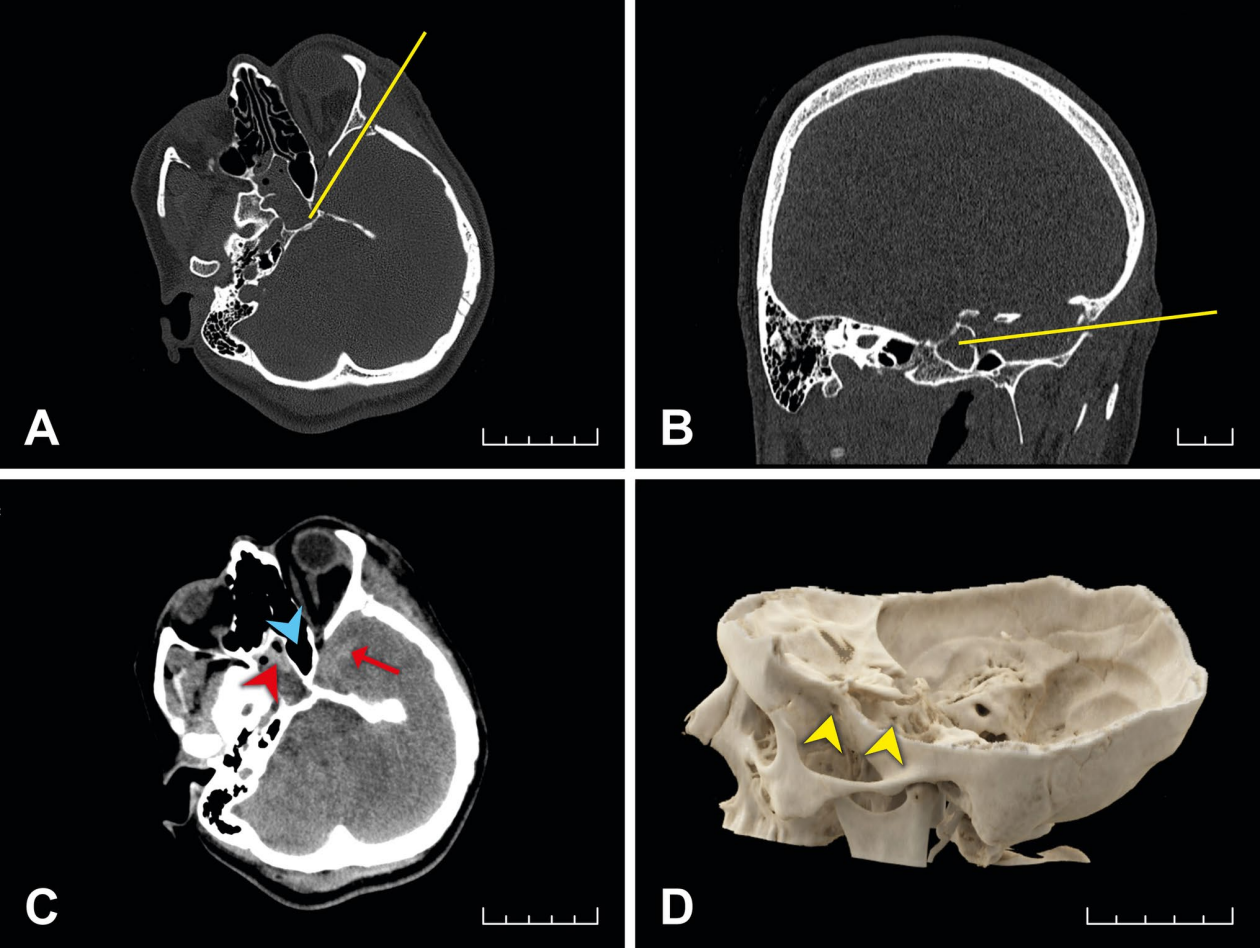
CT scan of the head in the axial and coronal plane displaying the stab track (yellow line in A and B, respectively). Blood in the right portion of the sphenoid sinus (red arrowhead in C), hypoplasia of the left portion of the sphenoid sinus with the presence of gas (blue arrowhead in C), blood in the stab track (arrow in C). The 3D reconstruction of the skull with penetration of the left temporal bone and sphenoid sinus (arrowheads in D)
